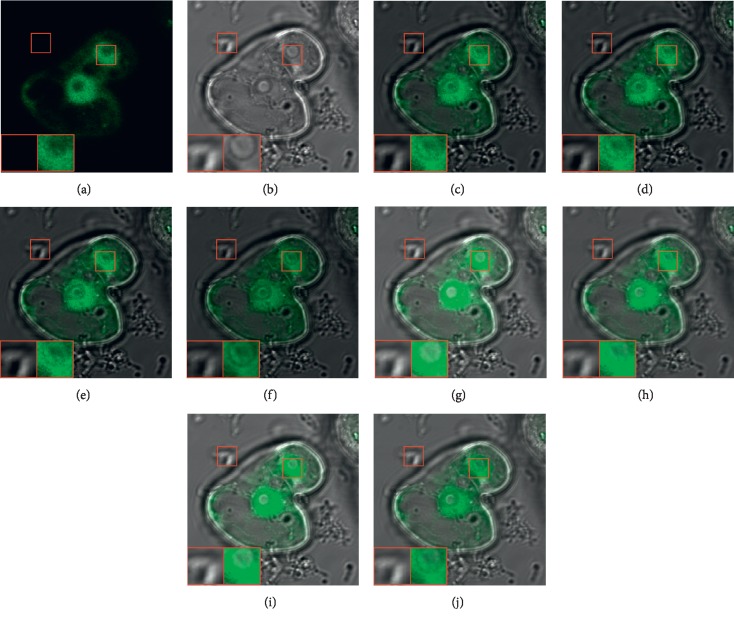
The first set of GFP and phase-contrast image fusion results. (a) GFP image. (b) Phase-contrast image. (c) DTCWT. (d) CVT. (e) NSCT. (f) SR. (g) CNN. (h) SFL-CT. (i) CST. (j) Proposed.
